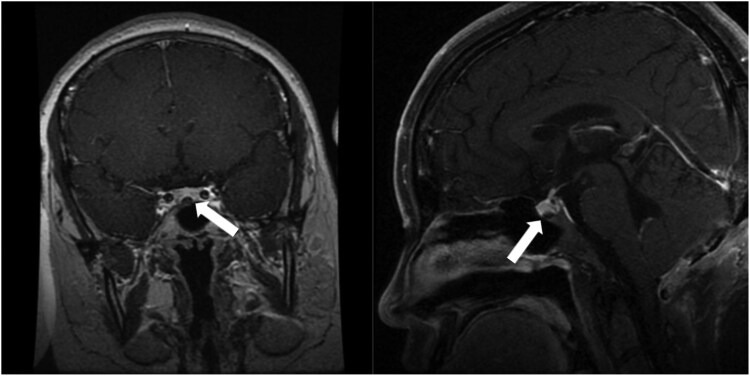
The magnetic resonance imaging (MRI) of the pituitary. The arrows show a decreased enhancement area in the midline area of the lower pituitary, with the possibility of microadenoma.
